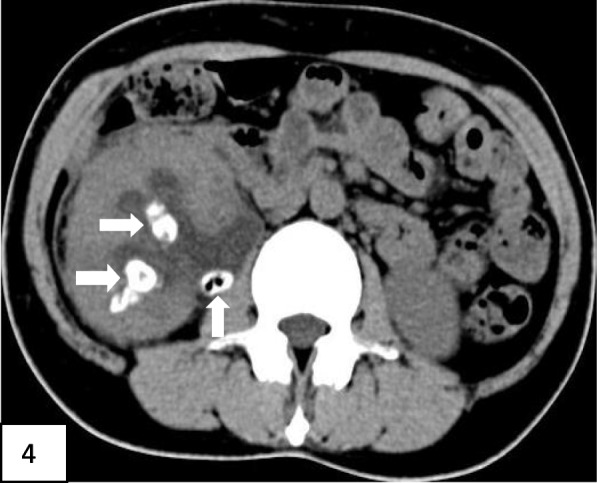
Shows the repeat non-contrast CT scan before hospital discharge
